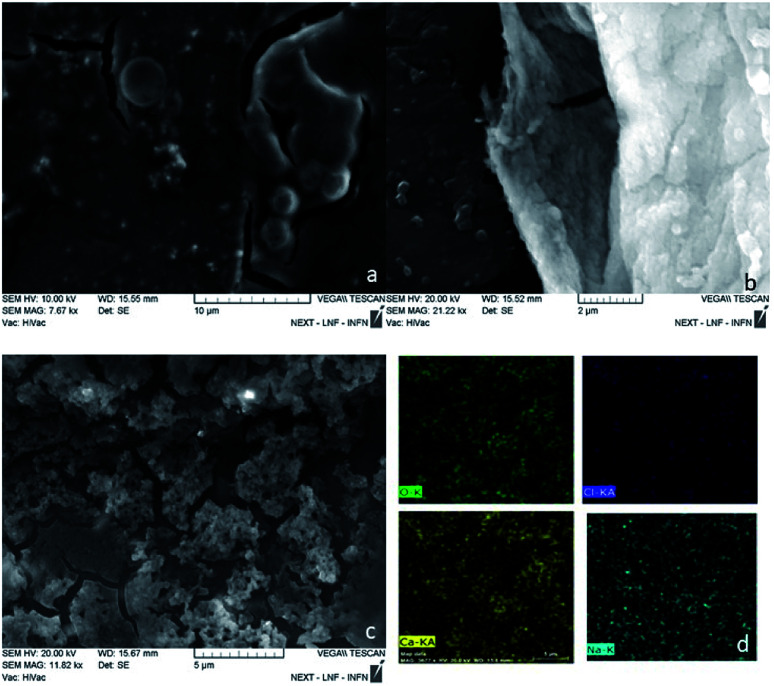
Effects of different top down setup on particle morphology the results obtained with (a) ultrasound tip, (b) homogenizer and (c) planetary mixer. In (d) the EDX mapping of panel (c) are reported on O–K (green), Cl-Kα (blue), Ca-Kα (yellow), Na–K (light blue).
